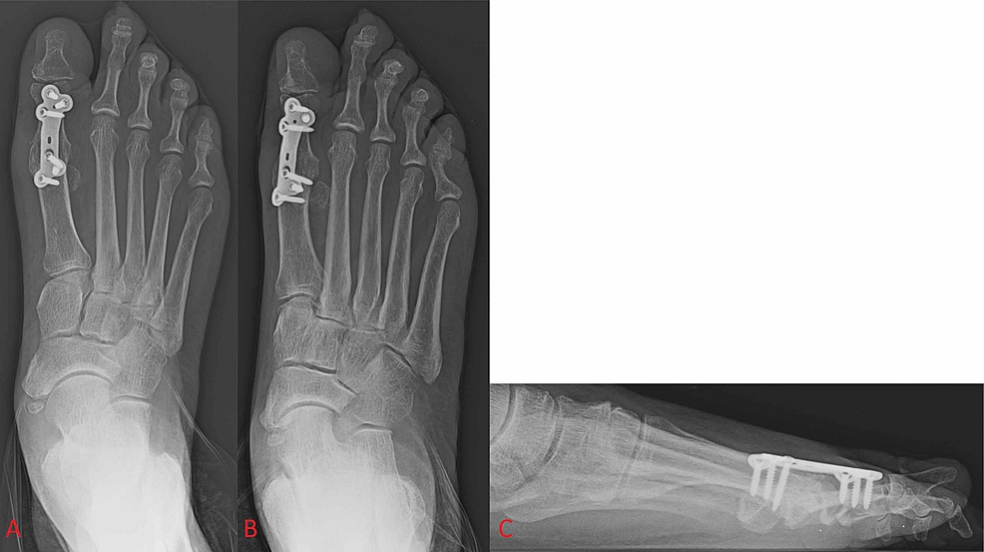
Foot X-ray shows dorsal locking plate fixation of the first metatarsophalangeal joint without a supplementary interfragmentary lag screw. Figure 4A and Figure 4B show anterior-posterior and oblique views of the foot, respectively. Figure 4C shows a lateral view of the foot.
